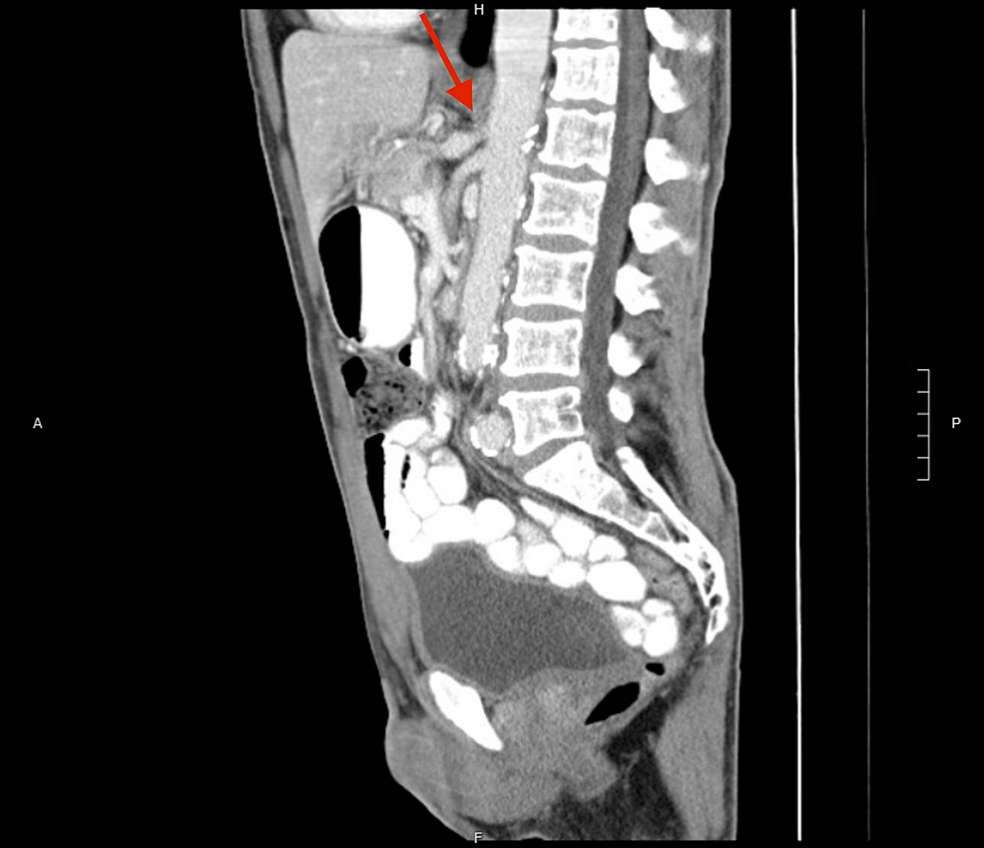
Preoperative CT of the abdomen/pelvis demonstrating celiac artery stenosis with post-stenotic dilatation consistent with MALS. CT: computed tomography; MALS: median arcuate ligament syndrome
